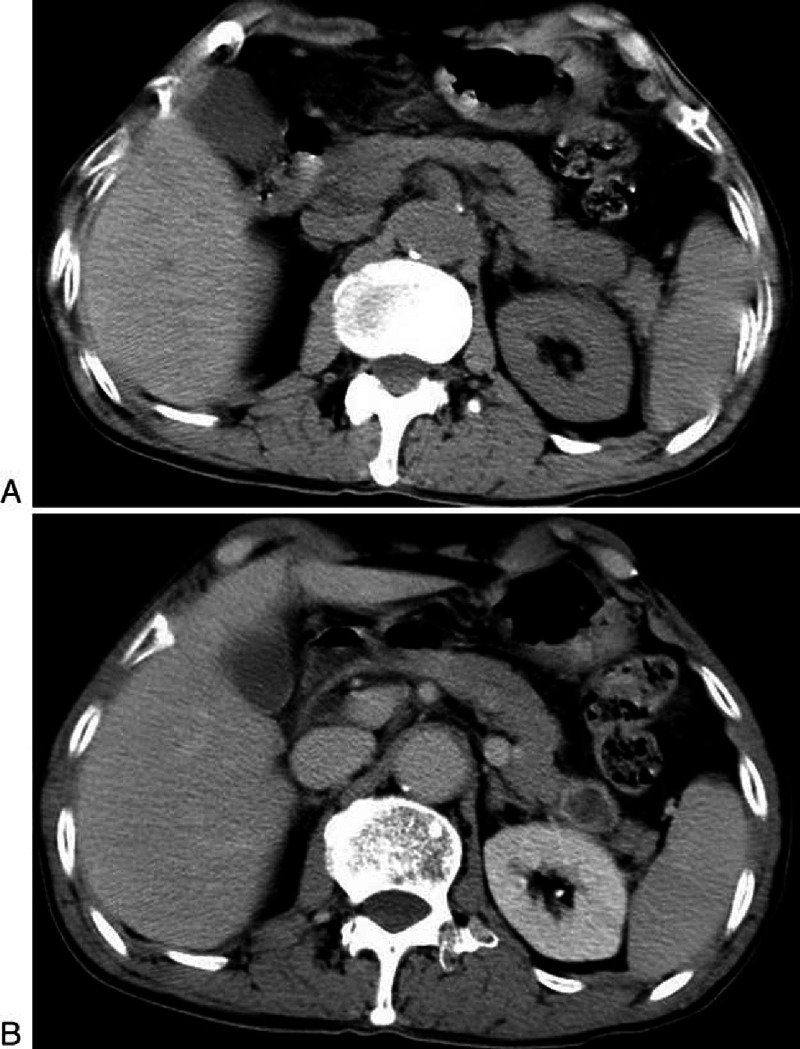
A 66-year-old man with NSCLC (patient 3). A, On unenhanced CT the density of metastases in pancreas are nearly identical with normal pancreatic parenchyma. B, Contrast enhanced CT show the lesion in the tail had characteristic peripheral enhancement.
